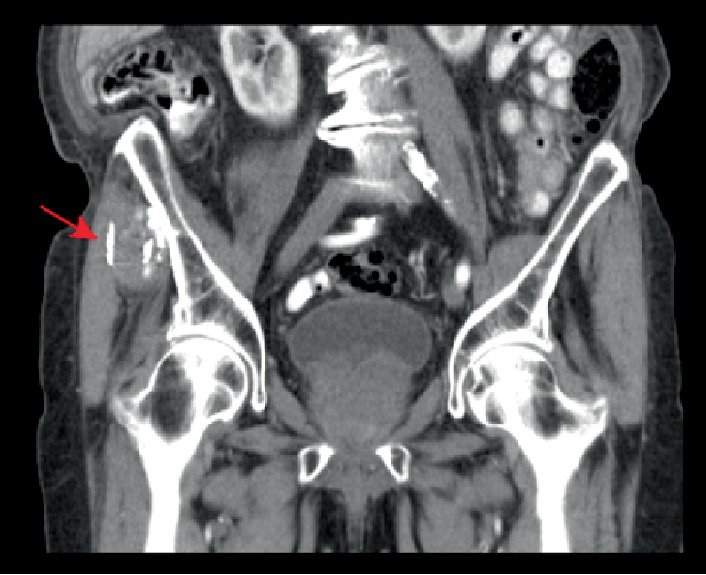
CT scan (coronal view) of the patient's abdomen and pelvis demonstrating radiopaque surgical material adjacent to the right ilium.
